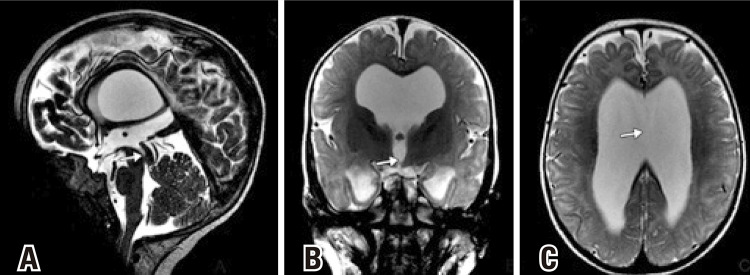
Magnetic resonance imaging T2-weighted sagital image. (A) Aqueductal web (arrow) with supratentorial hydrocephalus and ballooning of the chiasmatic recess. The corpus callosum was thin. Magnetic resonance imaging T2-weighted coronal image. (B) Axial image. (C) Dilatation of the lateral ventricles and of the third ventricle, septum pellucidum absence (arrow in C) and cerebral hypomyelination
